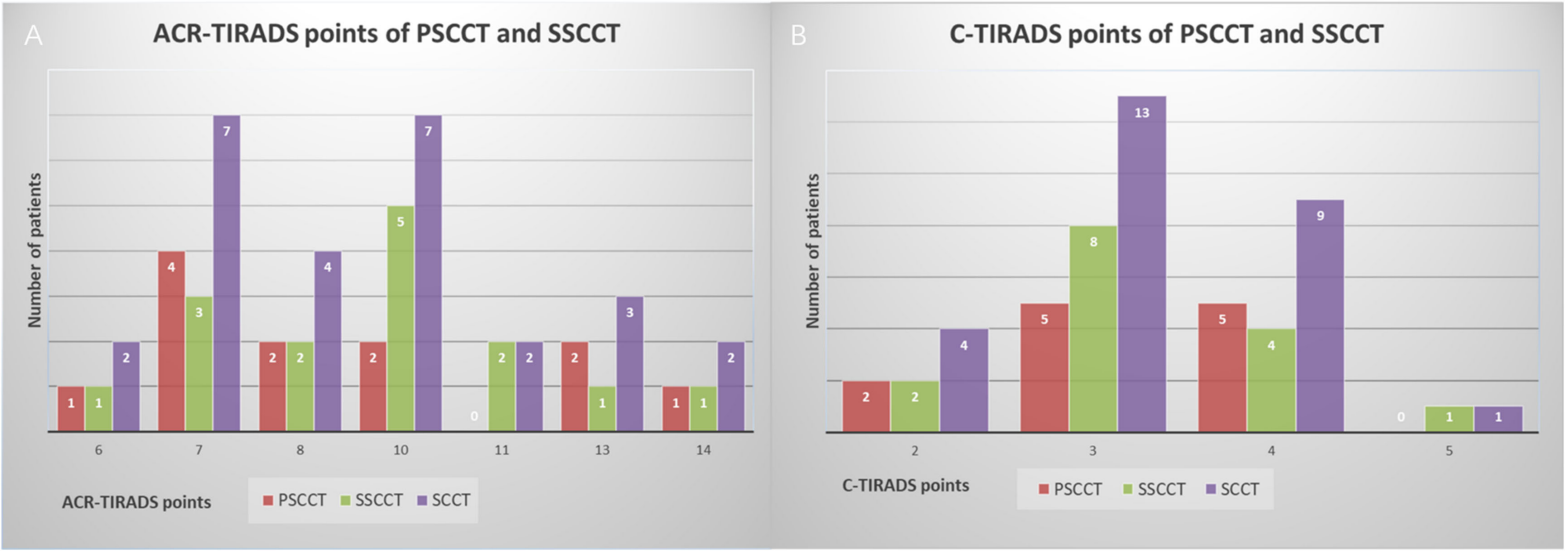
Points of SCCT (PSCCT, SSCCT) based on ultrasound in ACR-TIRADS (A) and C-TIRADS (B).
